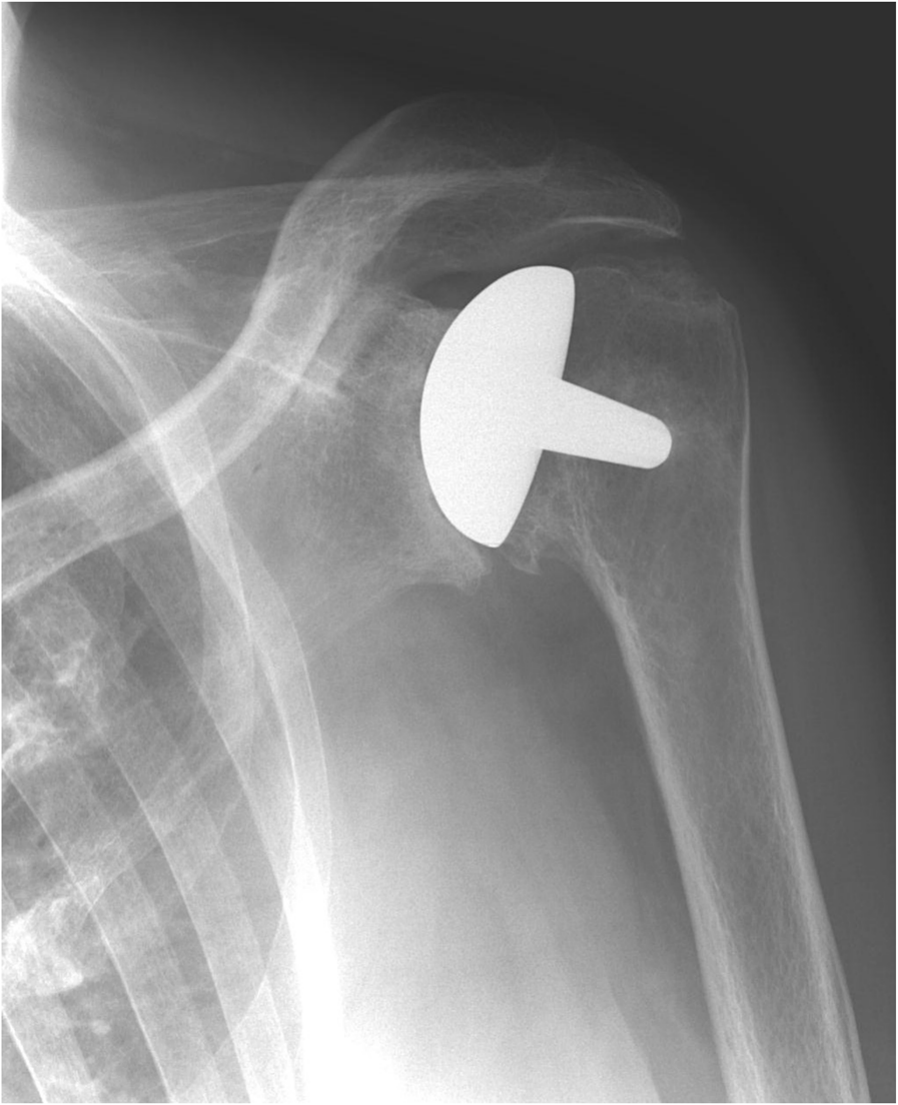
Post-implant AP radiograph where all observers classified the joint as overstuffed
